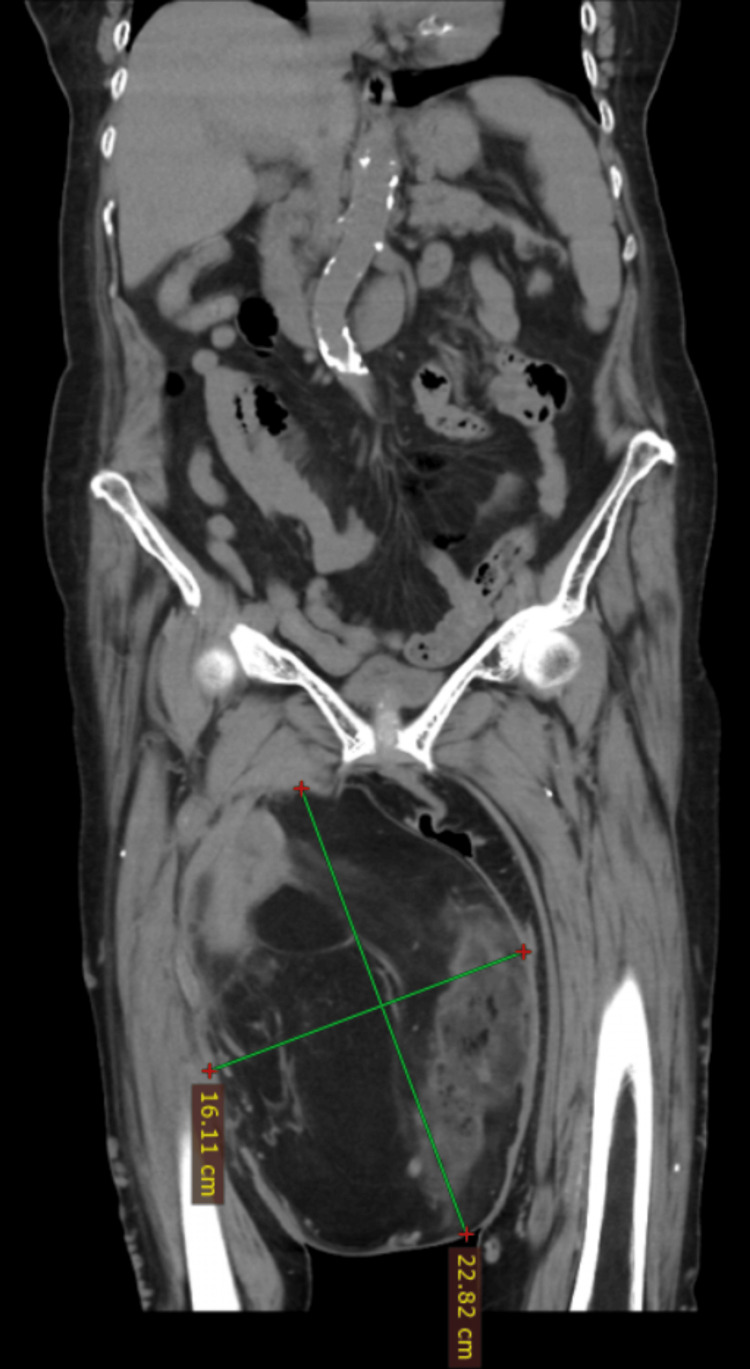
Coronal CT image showing a giant solid mass with areas of hypo- and hyperdensity.
